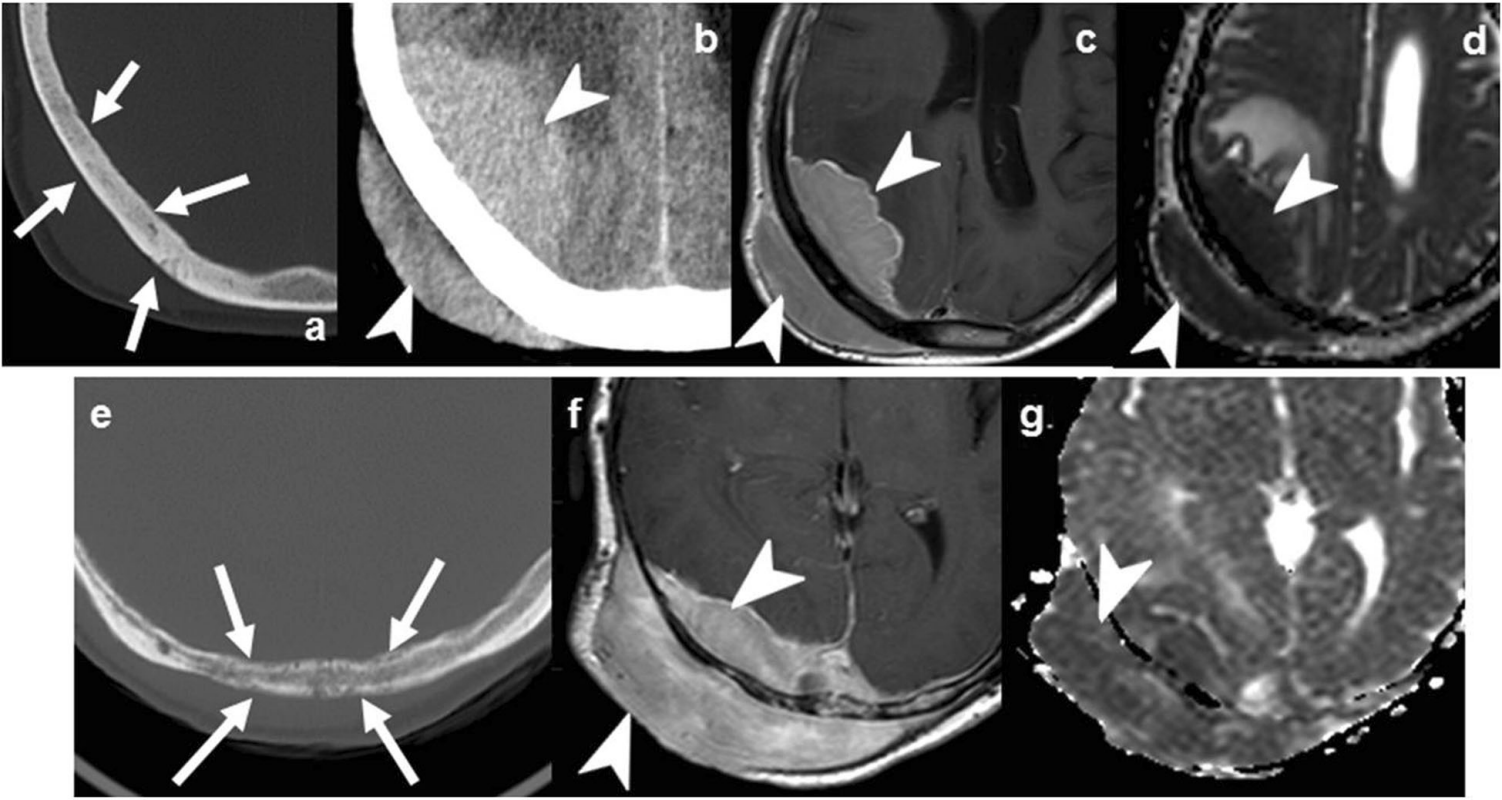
Lymphoma. Case 1 (a–d): NECT (a, b), post-contrast T1WI (c), and ADC map (d). Transdiploic mass with bone subtle sclerosis (arrows) and disproportionate soft-tissue components dense on CT, homogenously enhancing, and diffusion-restricting (arrowheads). Case 2 (e–g): Similar findings with bone lytic-permeation (arrows) instead
